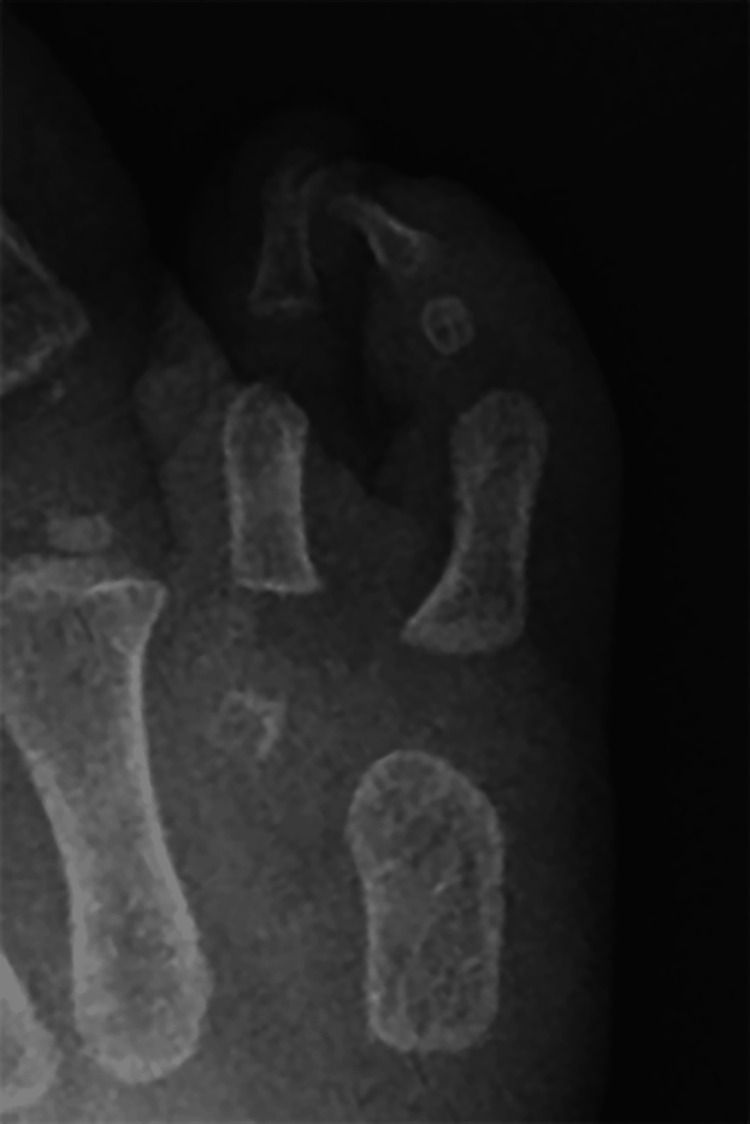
Radiographic image obtained before surgery
